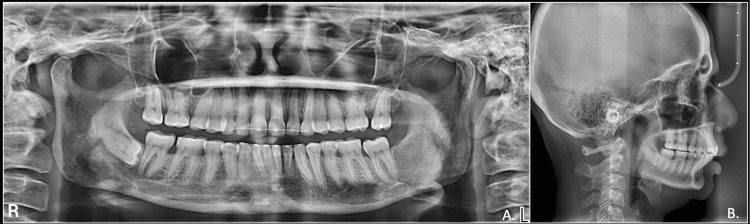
Post-treatment radiographs A: OPG; B: lateral cephalogram OPG: orthopantomogram
